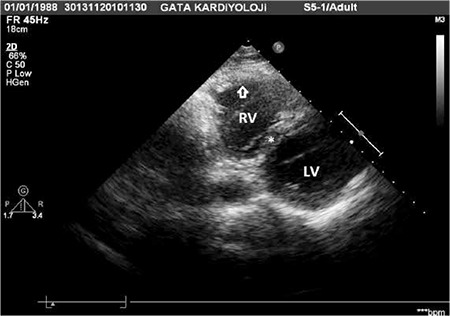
Transthoracic echocardiogram from left parasternal, basal, short axis projection also demonstrates hypertrophied [wall thickness (arrow): 11.4 mm] and dilated right ventricle (18.1 cm2). LV: Left ventricle, RV: Right ventricle, Asterisk: Interventricular septum.
